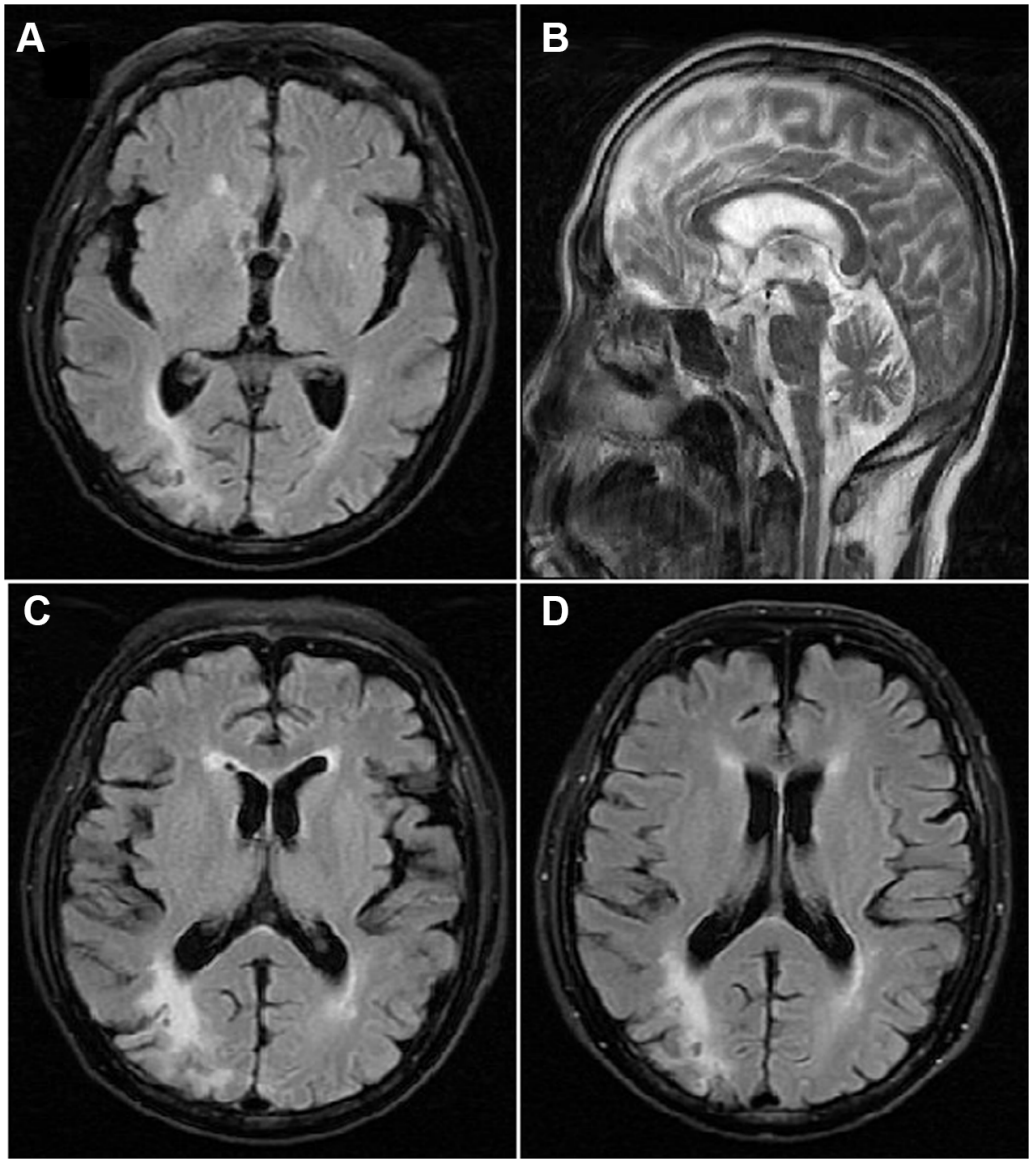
T2 weighted Axial view Magnetic resonance imaging showing (A) no infarct or space occupying lesion in the caudate nucleus, putamen or the globus pallidus, (B) and the third and fourth ventricle, and (C) encephalomalacia of the left frontal and right parietal lobes, gliosis, and signs of cerebral small-vessel disease; (D) encephalomalacia of the right parietal lobes at the second episode of chorea.
